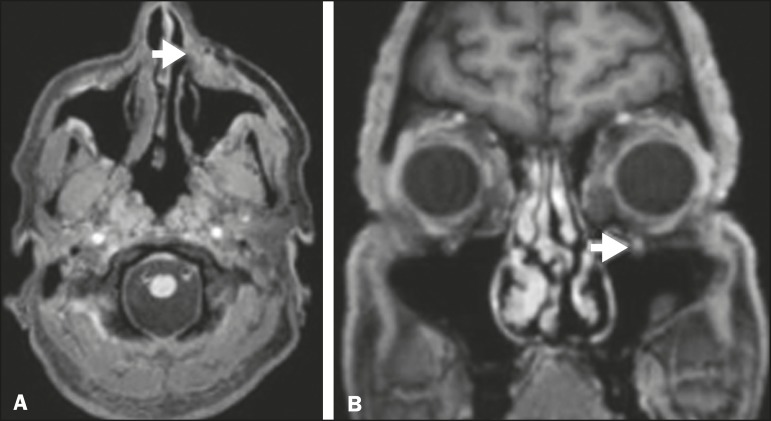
Gadolinium contrast-enhanced T1-weighted MRI scans of a 58-year-old man
